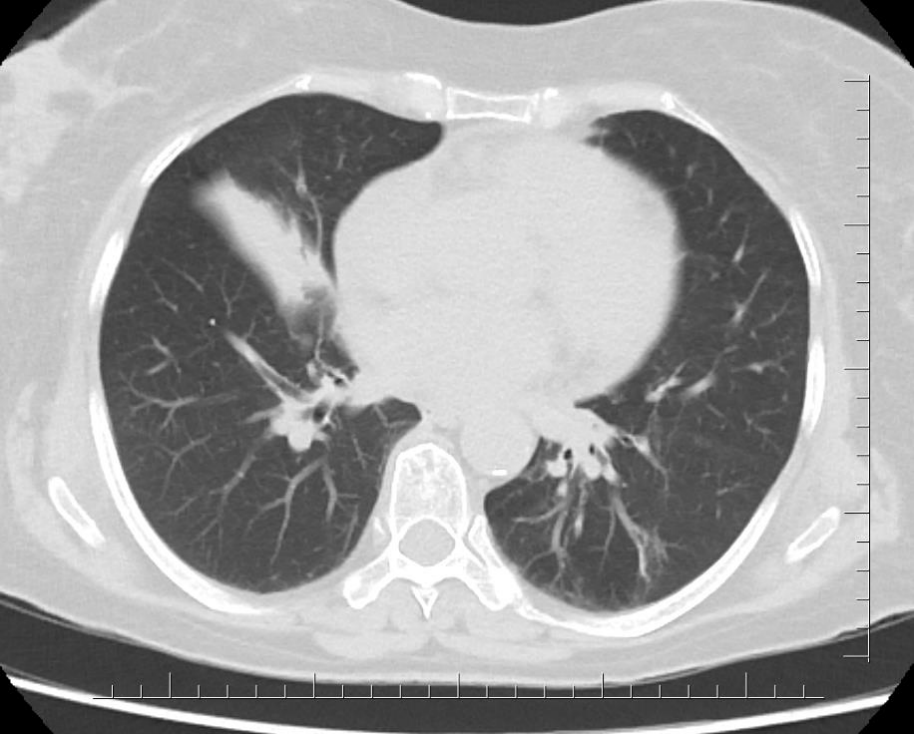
Chest CT-scan demonstrating lateral segmental collapse of the right middle lobe.
